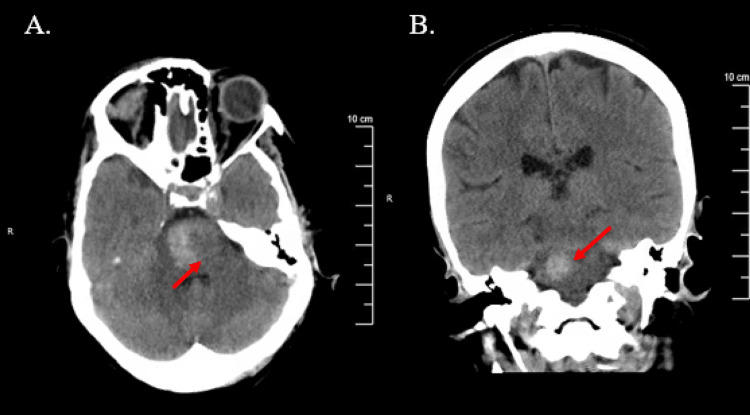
Non-contrast CT head axial and coronal views, respectively, showing the area of intracranial hemorrhage measuring 2.5 x 1.2 cm to the right side of the pons 24 hours after presentation in the ED. The patient still had significant neurological deficits at that time, but no change in severity.
